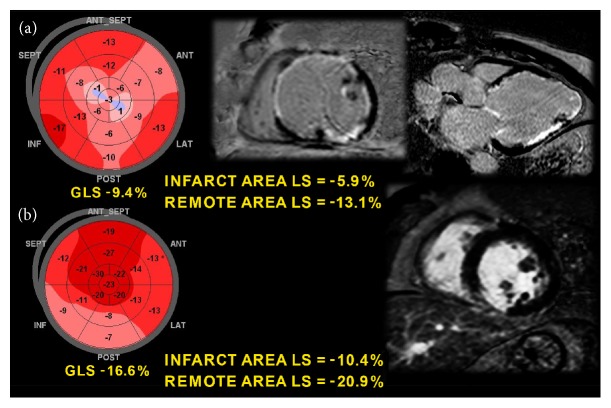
Comparison between global longitudinal strain and cardiac magnetic resonance imaging in two patients with (a) anterior myocardial infarction and (b) inferior myocardial infarction. The infarct areas of the bull's-eye map correspond closely with late gadolinium enhancement (scar) areas on magnetic resonance imaging.
